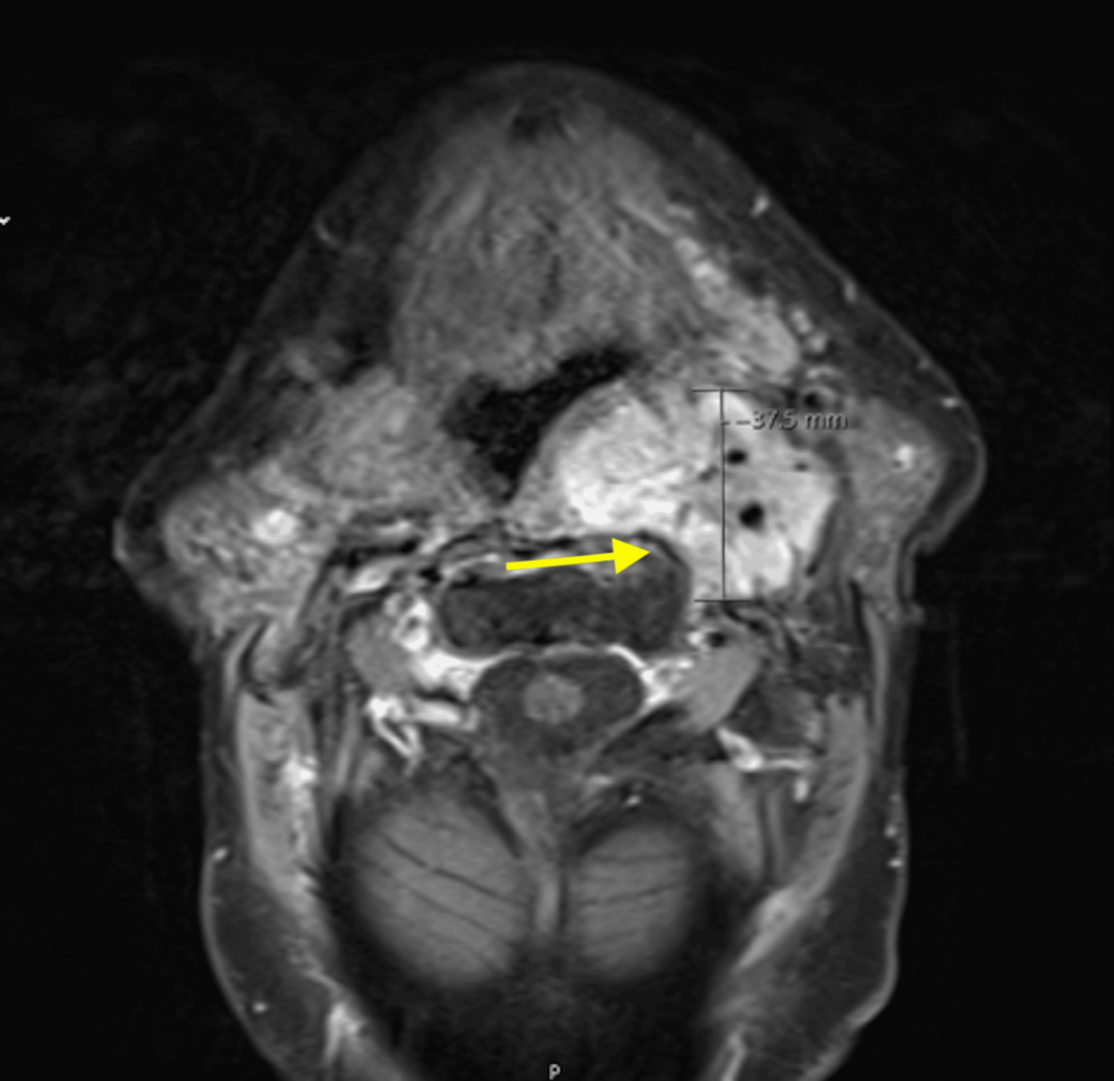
T1-weighted gadolinium-enhanced MRI sequence of the neck showing a mass on the left side (as indicated by the yellow arrow).
